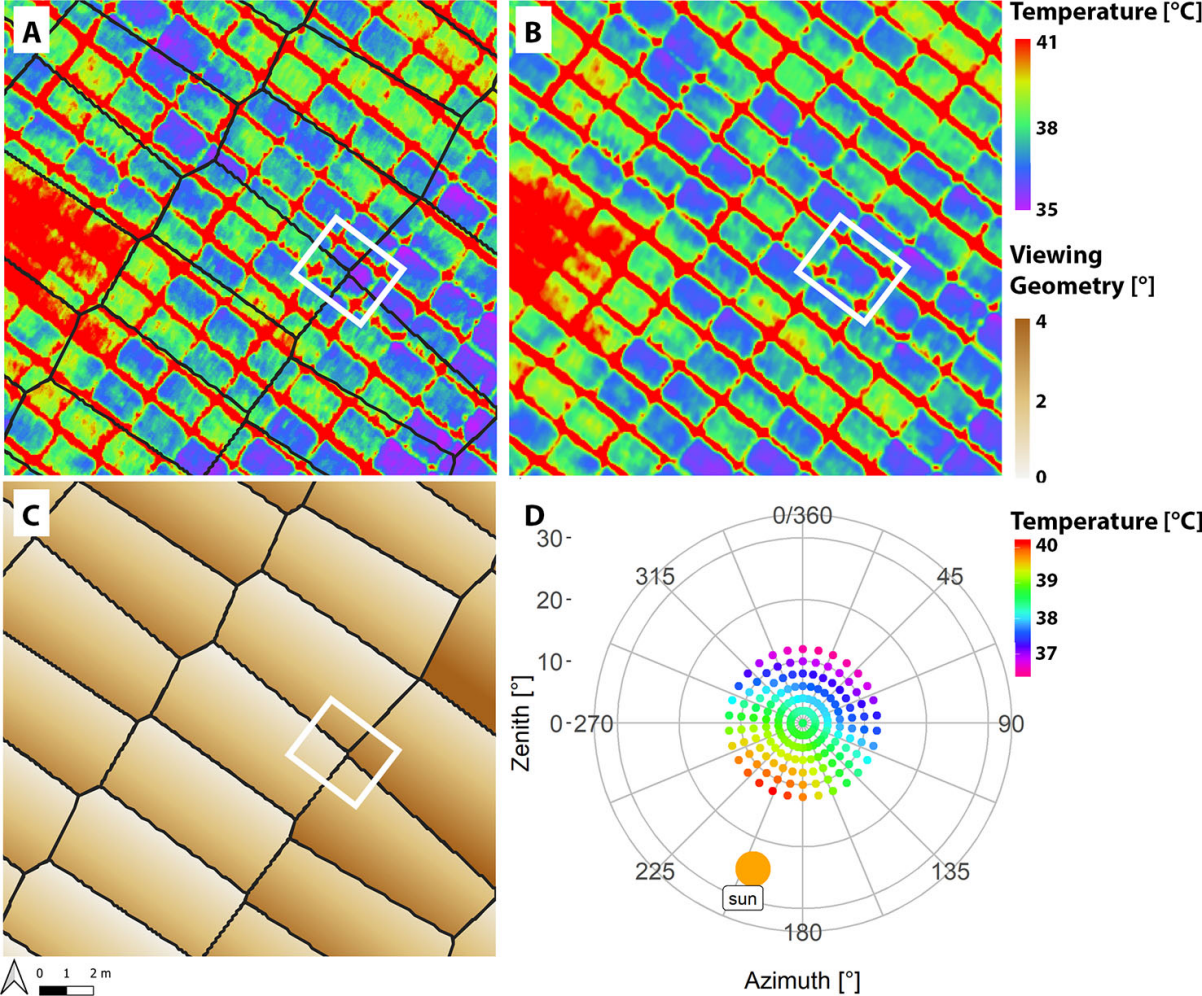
Excerpt of an orthomosaic generated with blending mode “disabled” (A) and “average” (B) on the 2018-06-20 at 14:00 h local time. The color corresponds to the apparent canopy temperature (CT). (C) shows the viewing geometry of the information used to generate the blending mode “disabled” orthomosaic (A). In A and C, black seamlines mark the border of the information that is taken from different images. The white box highlights a plot where information from four images are composed in the blending mode “disabled” mosaic (A). (D) shows the average apparent temperature of all plots of that flight in dependence of its viewing geometry. The sun had an azimuth angle of approx. 199° and a zenith angle of approx. 25°. The viewing geometry of the apparent temperature was calculated from the relative position of the camera seen from the plot.
